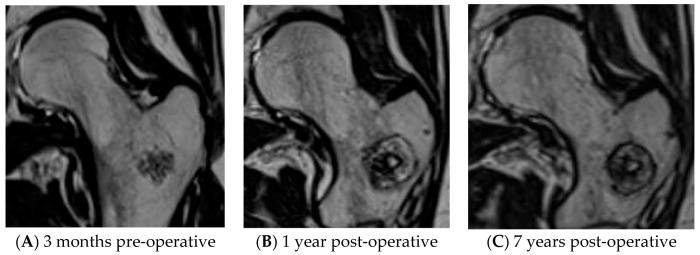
Low-grade cartilaginous tumor in femur before and after RFA treatment. (A) ACT on pre-operative MRI. (B) ACT on MRI, 1 year after RFA. (C) ACT on MRI, 7 years after RFA.
